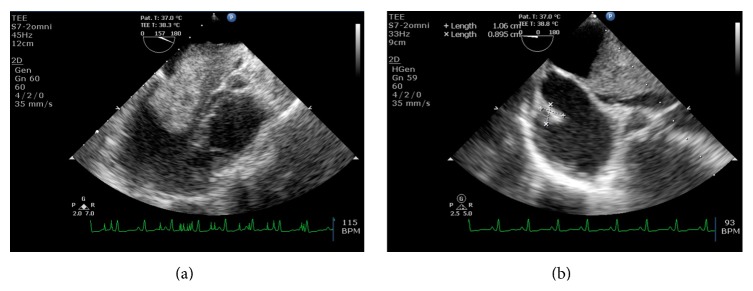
2D transesophageal echocardiogram revealed (a) large left atrial mass of 6.6 × 3.1 cm size and (b) right atrial mass of 1 × 1 cm size.
